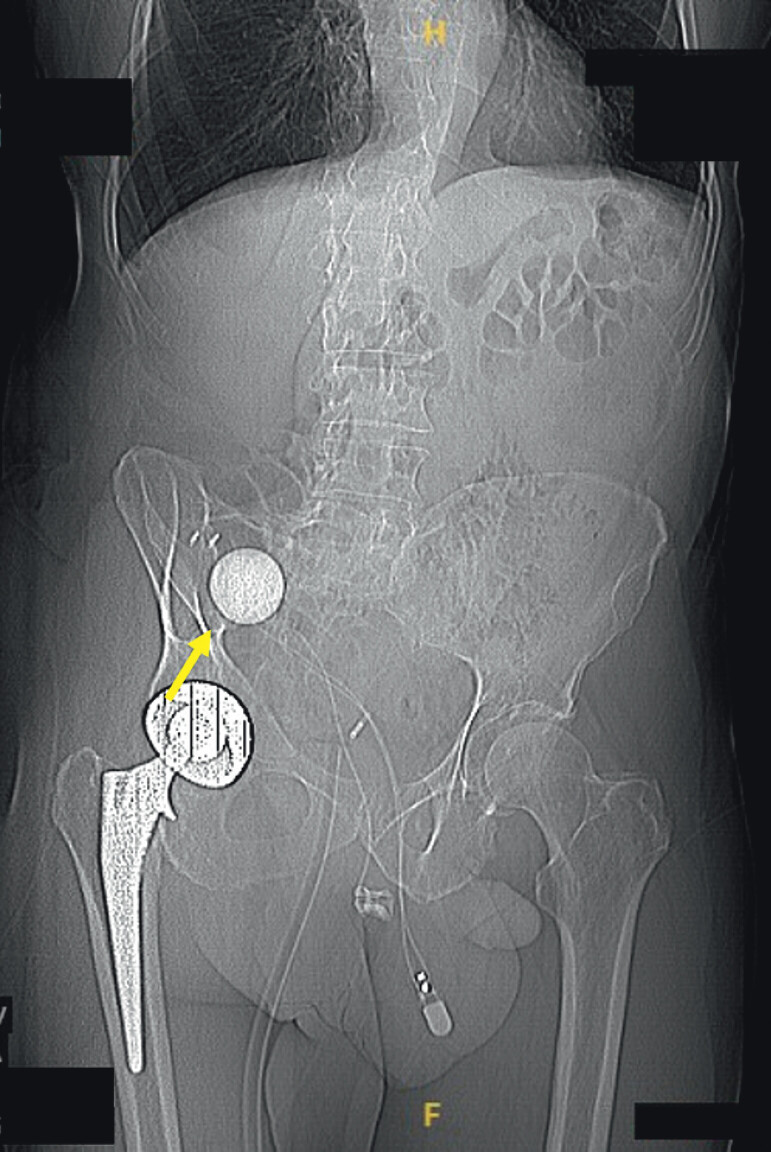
Computed tomography scan showing an inflatable penile prosthesis, with the fluid reservoir located in the right lower quadrant, directly abutting the cecal wall.
